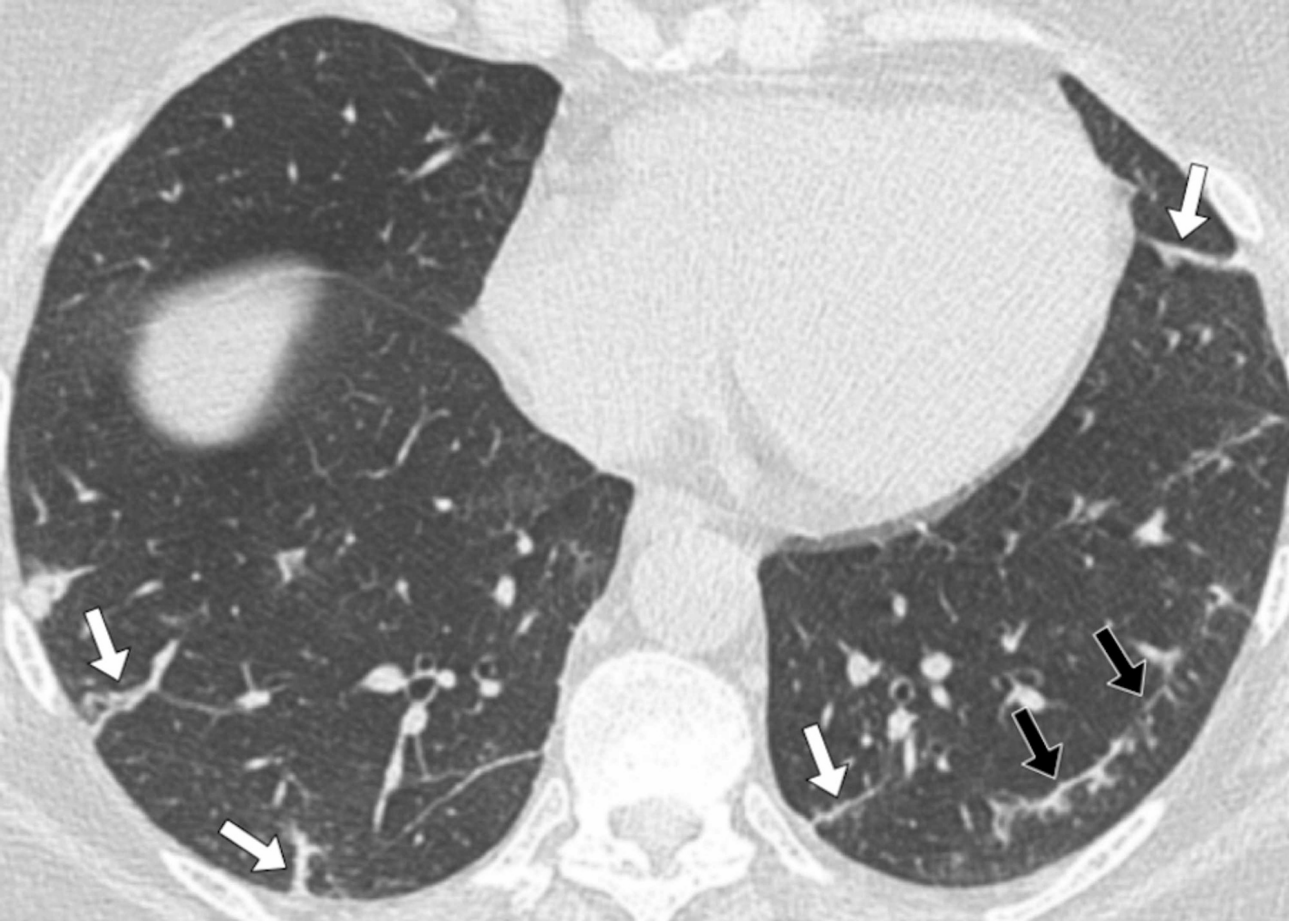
Patient 5 axial CT image An axial computed tomography (CT) image from the same study, at the level of the lung bases, demonstrates multiple thickened and irregular septae and a curvilinear subpleural line (black arrows). These features are commonly seen in COVID-19 patients.
